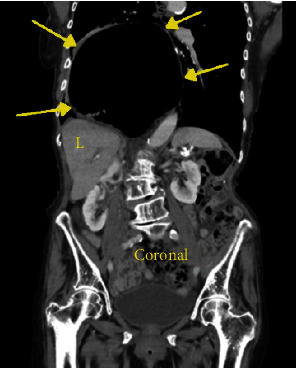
Coronal CT of chest and abdomen showing the large hiatal hernia located above the diaphragm with the liver “L” apparent.
